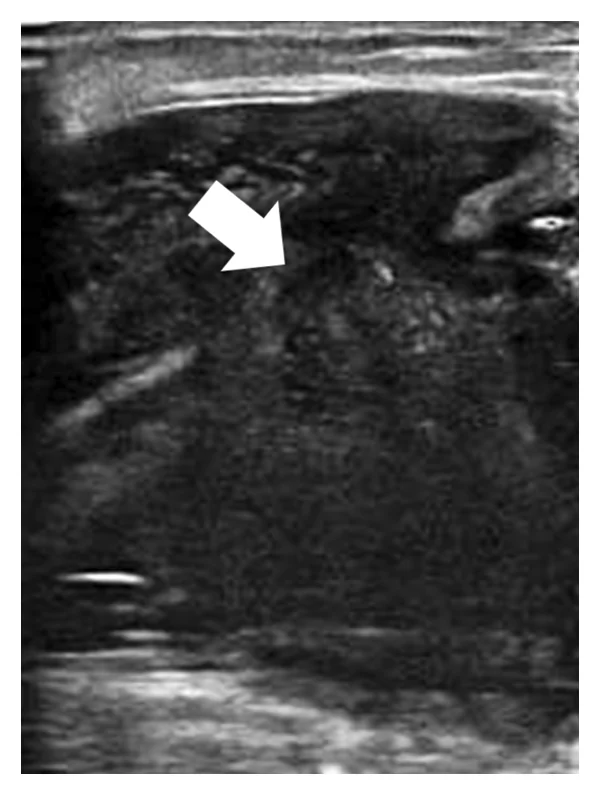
Abdominal ultrasonography demonstrating an abdominal wall abscess with a fistulous tract extending through the abdominal wall musculature and communicating with the peritoneal cavity (arrow).
